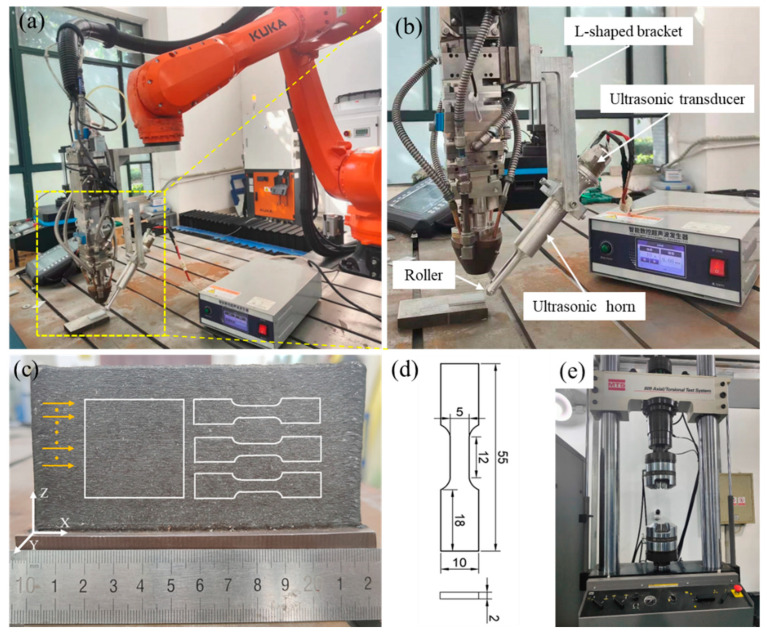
(a) LDED-UR device, (b) detailed structure of the ultrasonic rolling setup, (c) the manufactured thin-wall structure, (d) the size of the fatigue sample, and (e) fatigue testing machine.
